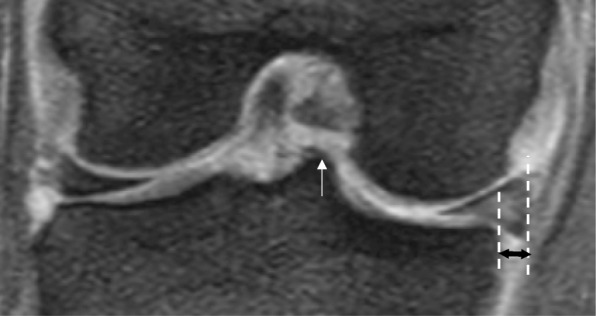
Magnetic resonance image-based measurement of medial meniscus extrusion (MME). Measurement of MME is performed using a bony landmark method. On the coronal plane, the medial tibial eminence (*white arrow*) is used to measure the horizontal distance between the most medial aspect of the tibia and the most medial aspect of the meniscus (*black double-headed arrow*)
